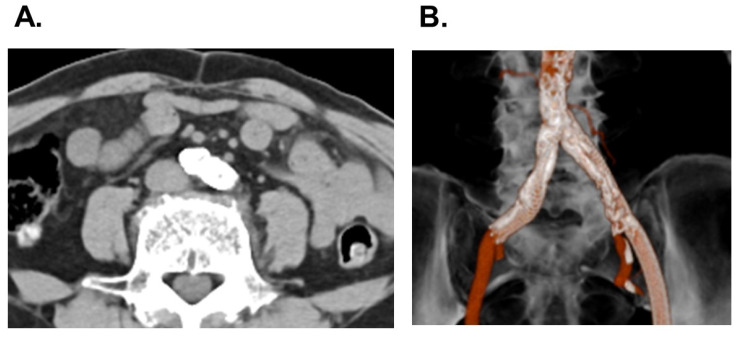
Abdominal computed tomography as a horizontal view (A) and three-dimensional view (B) on admission, possessing bilateral common iliac stents with small in-stent lumens.
